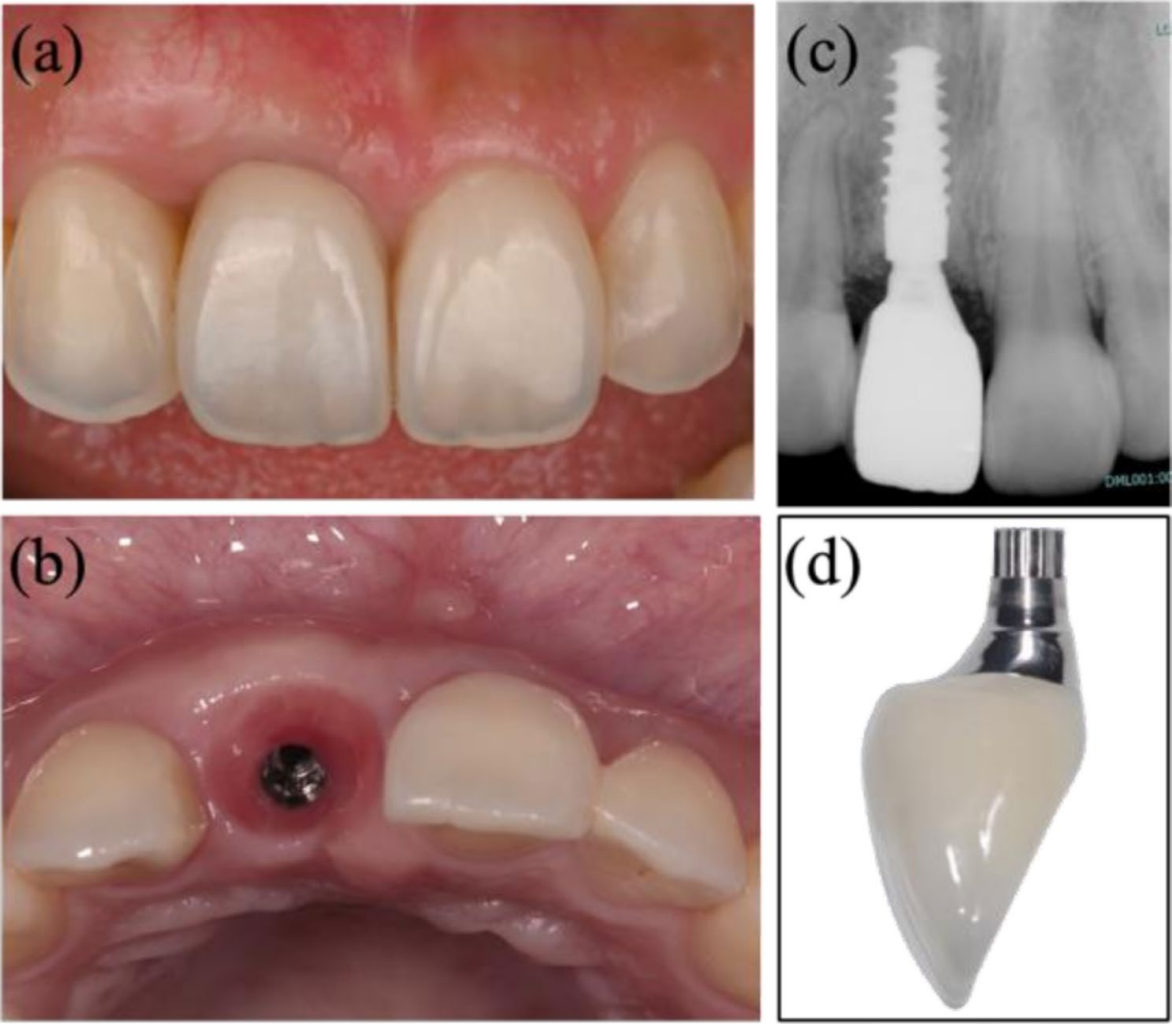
Implant-supported crown in harmony with adjacent maxillary right central incisor. Considering the subgingival contour distance and applying a concave subgingival contour allowed the thickness of the labial tissue to be maintained and soft tissue recession was suppressed. (a) Frontal view; (b) occlusal view; (c) dental X-ray; (d) superstructure
